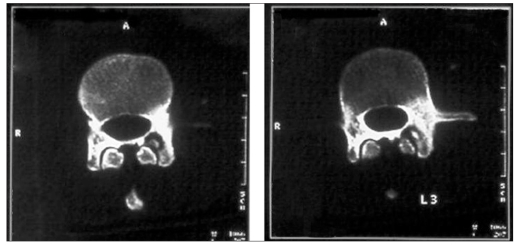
CT scan confirming osteoid osteoma in left pedicle with classical central sclerotic nidus
